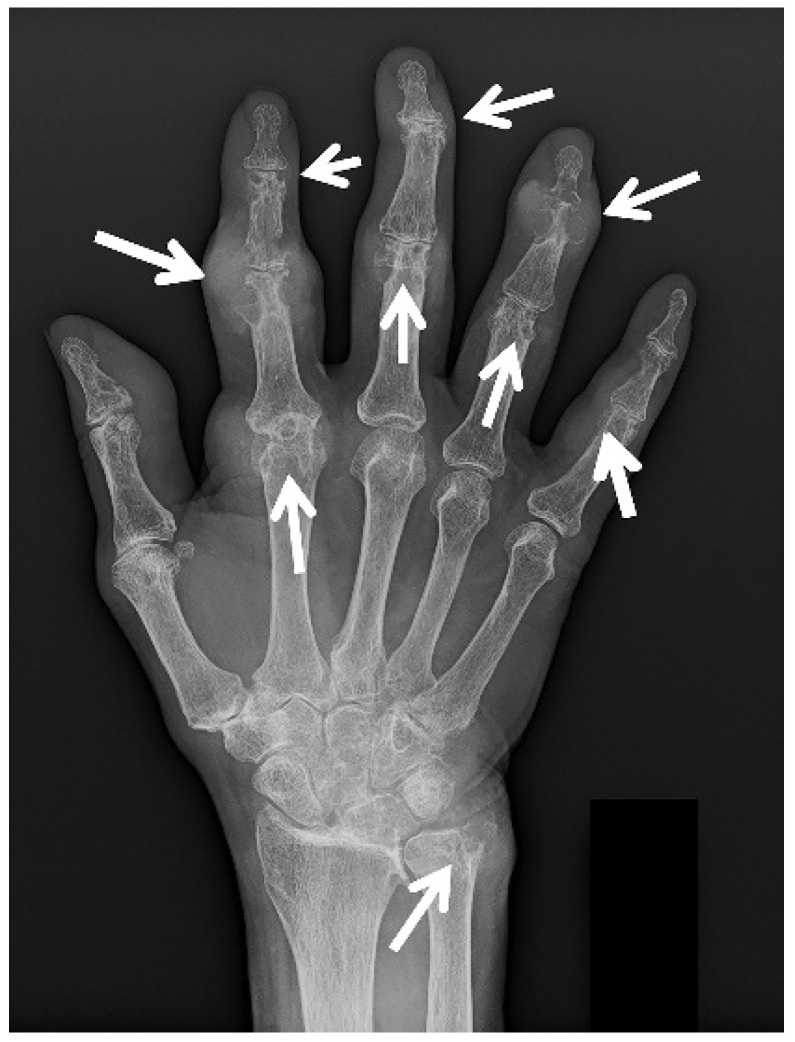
A 74-year-old man with gouty arthropathy involving bilateral hands. PA radiograph of the right hand shows erosive and cyst-like changes about multiple joints of the hand and ulnar styloid with adjacent dense soft-tissue nodules (arrows) consistent with gouty arthropathy. Several erosions have overhanging edges, most notable at the radial aspect of the index finger proximal interphalangeal joint. Note faint calcifications within the nodular thickening adjacent to the ulnar styloid erosion.
